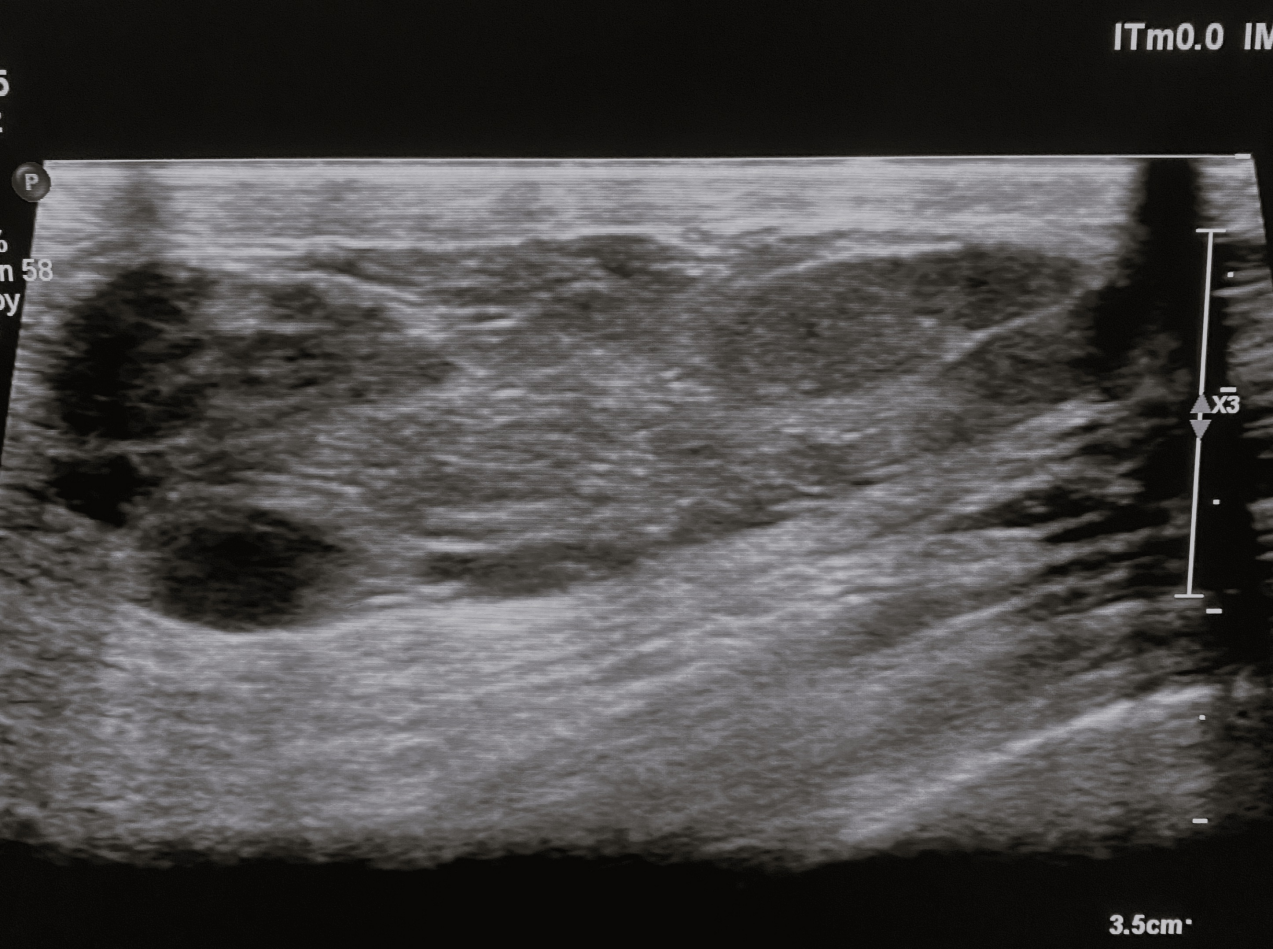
Breast ultrasound Breast ultrasound shows a tissue formation with the long axis parallel to the cutaneous plane, well-circumscribed, heterogeneous, with hypoechoic with hyperechoic trabeculae and posterior acoustic enhancement, designated as BI-RADS 2.
